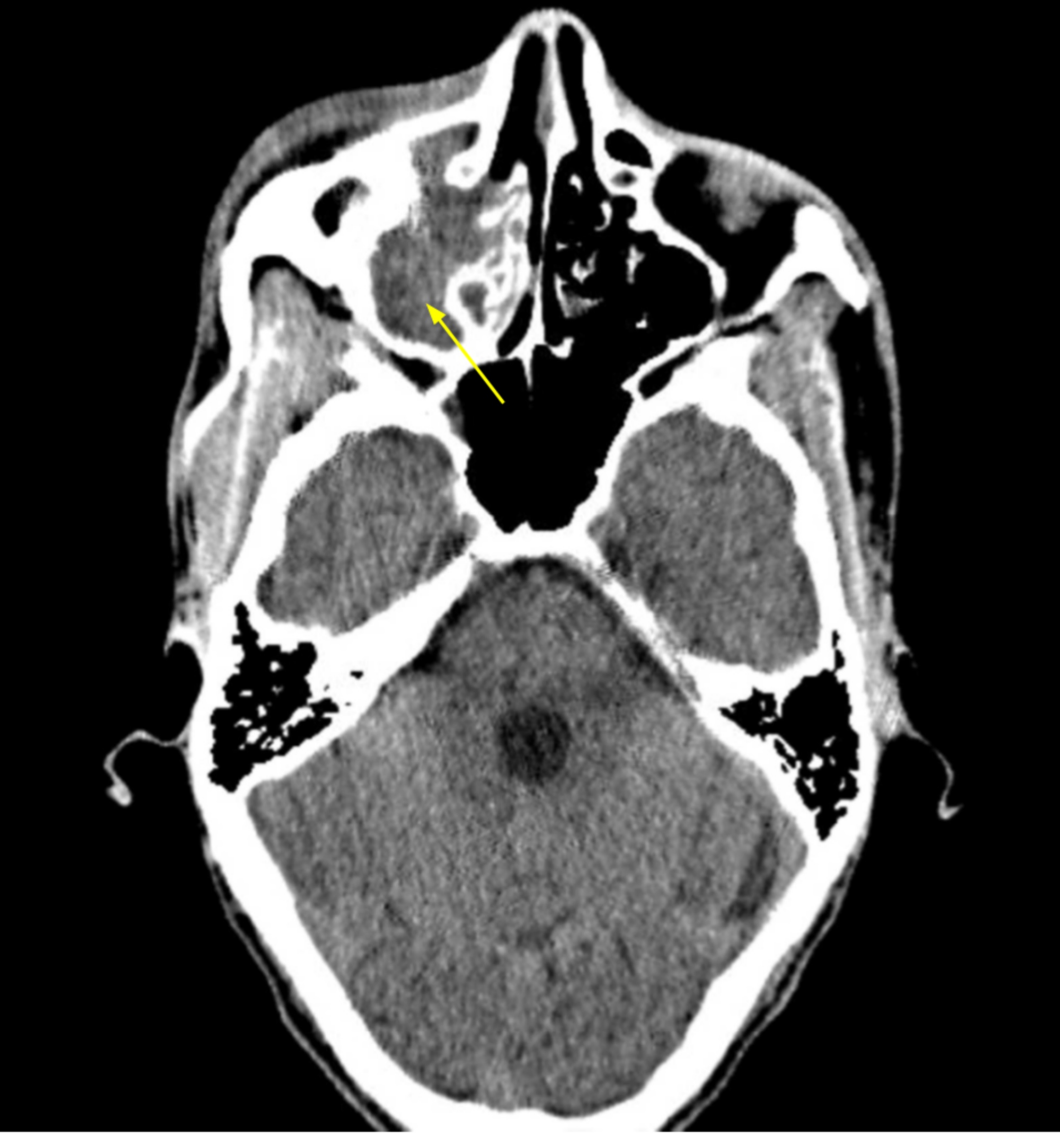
Opacification of the right maxillary sinus on computed tomography of the head.
